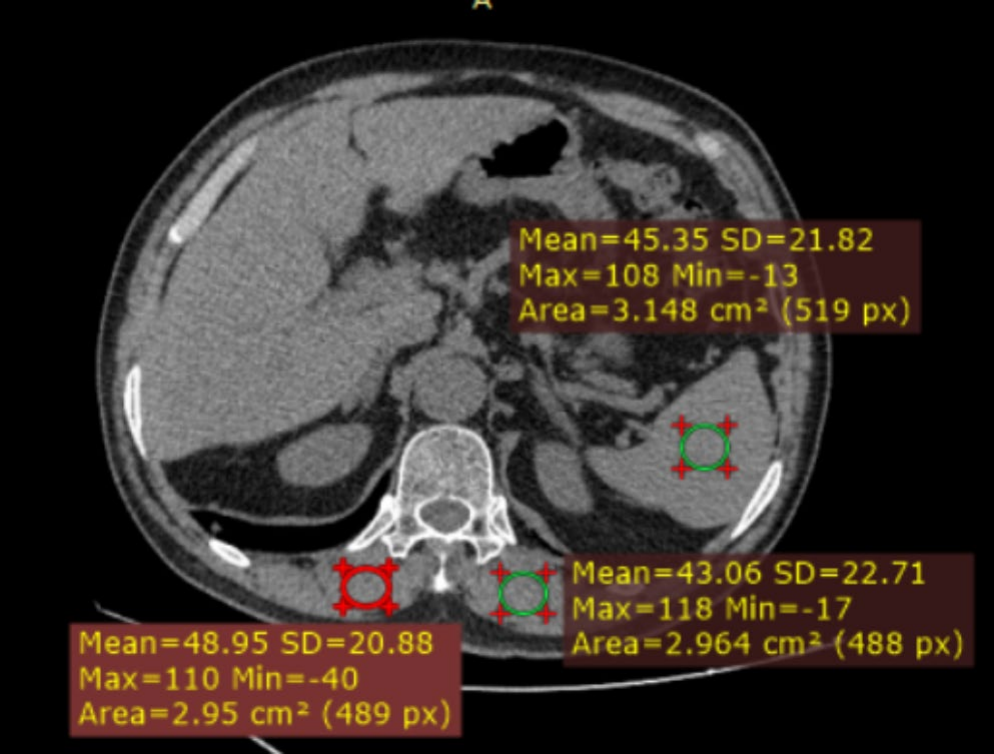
Measurement of muscle CT attenuation. A: Axial computed tomography image at the T11 level. B: Muscular and spleen CT attenuation was measured with a region of interest of 2.0 cm^2^
